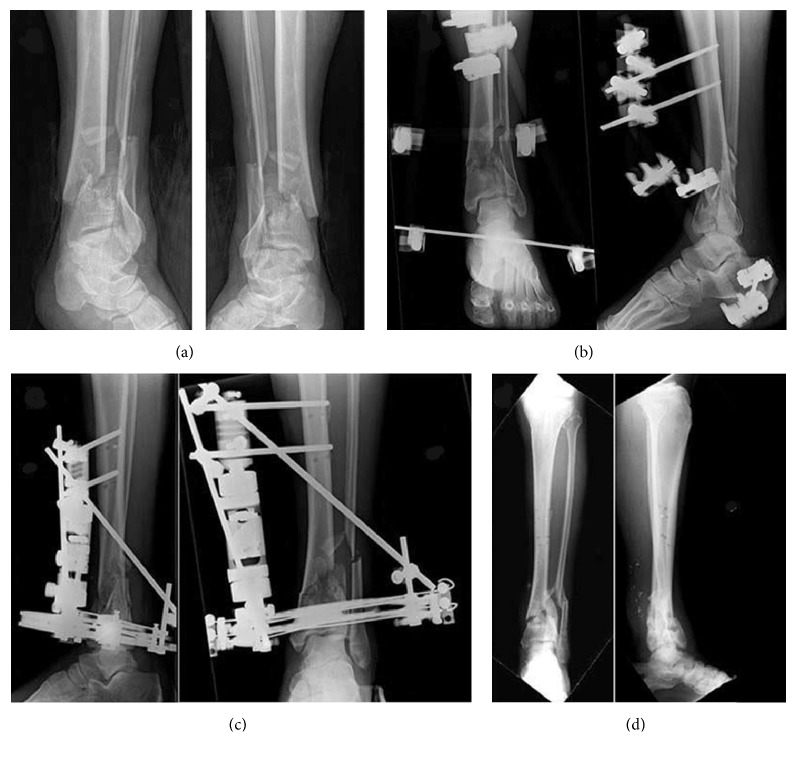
Case N*°* (34) of Table 2:* a 43-year-old man with a 43-C1 open fracture (Gustilo II) treated with* hybrid external fixator:* (a) preoperative radiographic image; (b) after prefix implant, radiographic image; (c) definitive EF implant after 1 week, radiographic image; (d) radiographic aspect at 57-month follow-up after implant removal.
